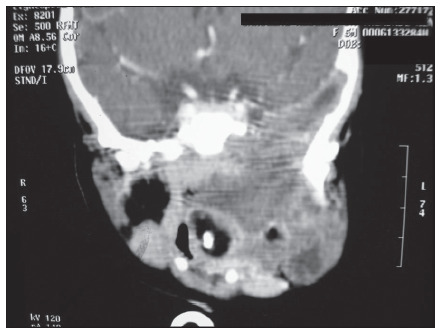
Neuroglial heterotopia shown by magnetic resonance imaging.
